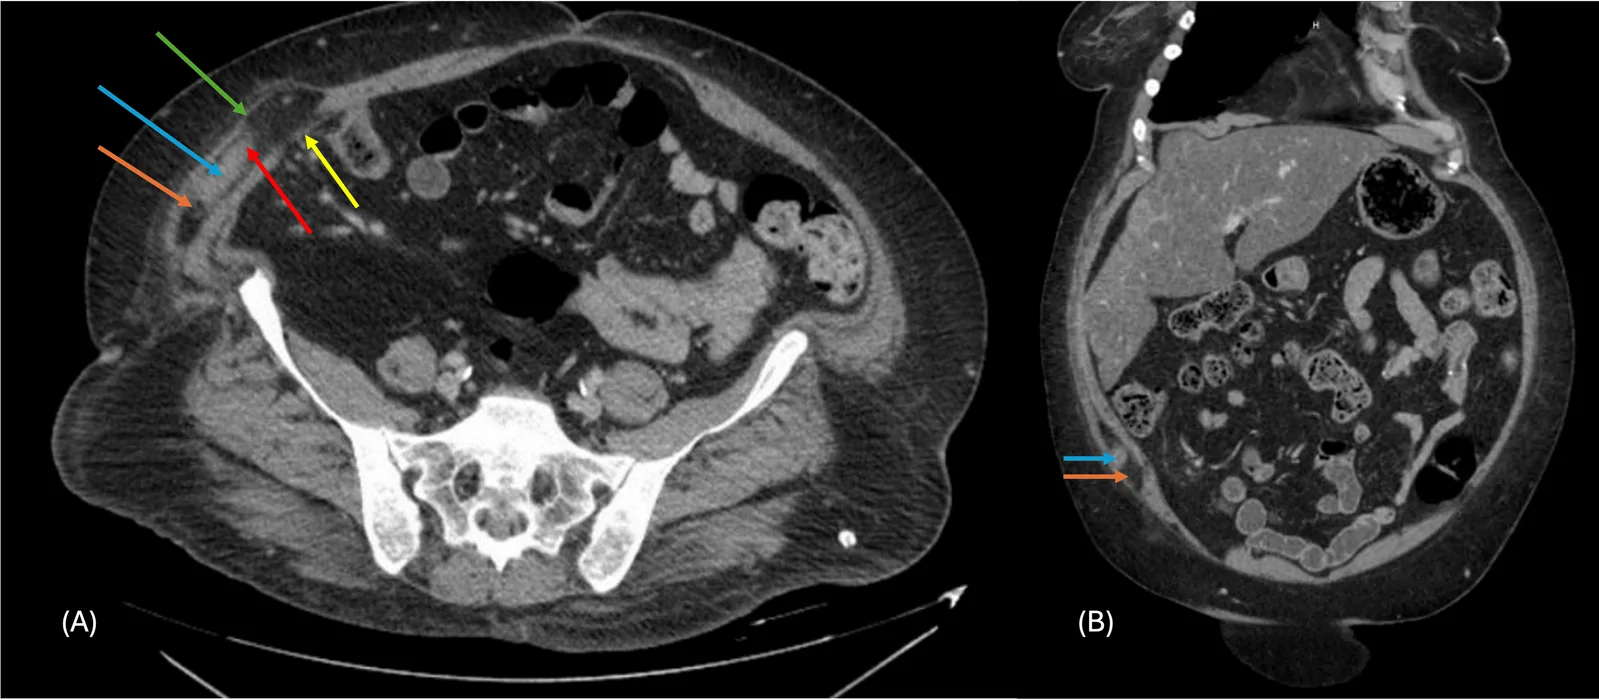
Preoperative CT of the Abdomen and Pelvis with Contrast CT images demonstrating acute appendicitis within a right lower lateral abdominal wall Spigelian hernia. Axial image (A) demonstrates an inflamed appendix (blue arrow) contained within the hernial sac (green arrow). The fascial defect (yellow arrow) and hernia neck (red arrow) are identified at the site of protrusion through the abdominal wall. Surrounding periappendiceal inflammatory fat stranding (orange arrow) is present, consistent with acute appendicitis. Sagittal image (B) further demonstrates the inflamed appendix (blue arrow) with adjacent inflammatory fat stranding (orange arrow) extending within the hernia sac.
